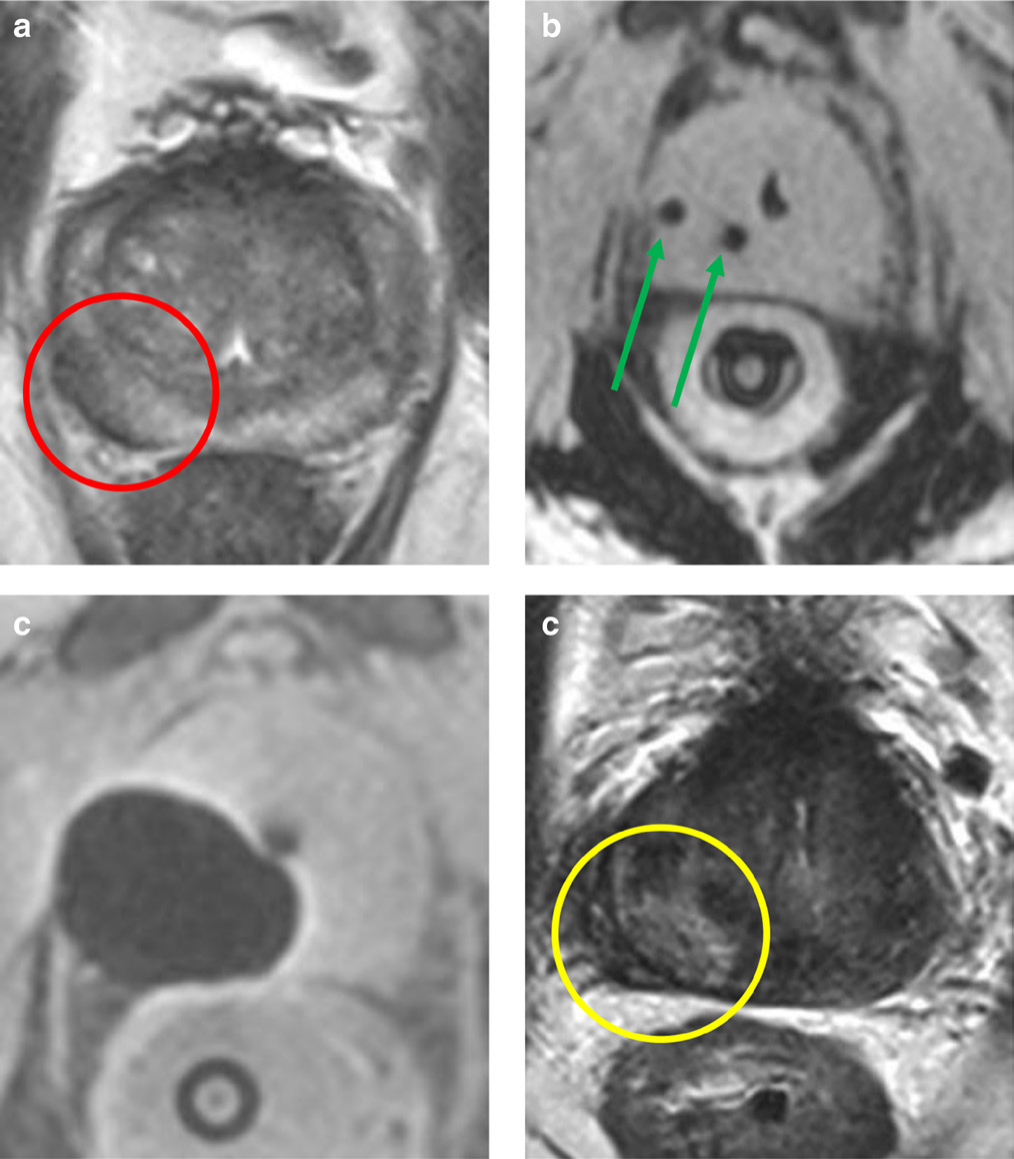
Cryoablation MRI of a 77-year-old male with an initial PSA of 11.4 ng ml^−1^ that underwent EBRT in 2012 as treatment for a *de novo* lesion (Gleason score 4+4=8) at the right peripheral zone. During follow-up, the serum PSA levels increased from 1.1 to 2.7 ng/ml. Multiparametric MRI demonstrated a recurrent lesion at the right peripheral zone (PI-RADS 4). Patient underwent cryotherapy for a recurrent Gleason score 4+5=9 prostate tumor. (a) Axial *T*
